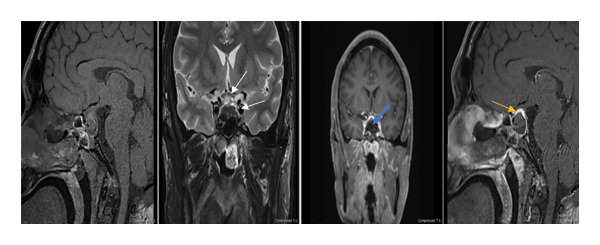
MRI pituitary with contrast (postoperative). Postoperative selective MRI images of the pituitary region (sagittal T1WI, coronal T2WI, and post‐IV contrast coronal and sagittal images) show an empty sellar and suprasellar fossa with reduced compressive effects on the chiasm and carotids (white arrow), peripheral enhancement (yellow arrow), and no soft tissue component (blue arrow).
